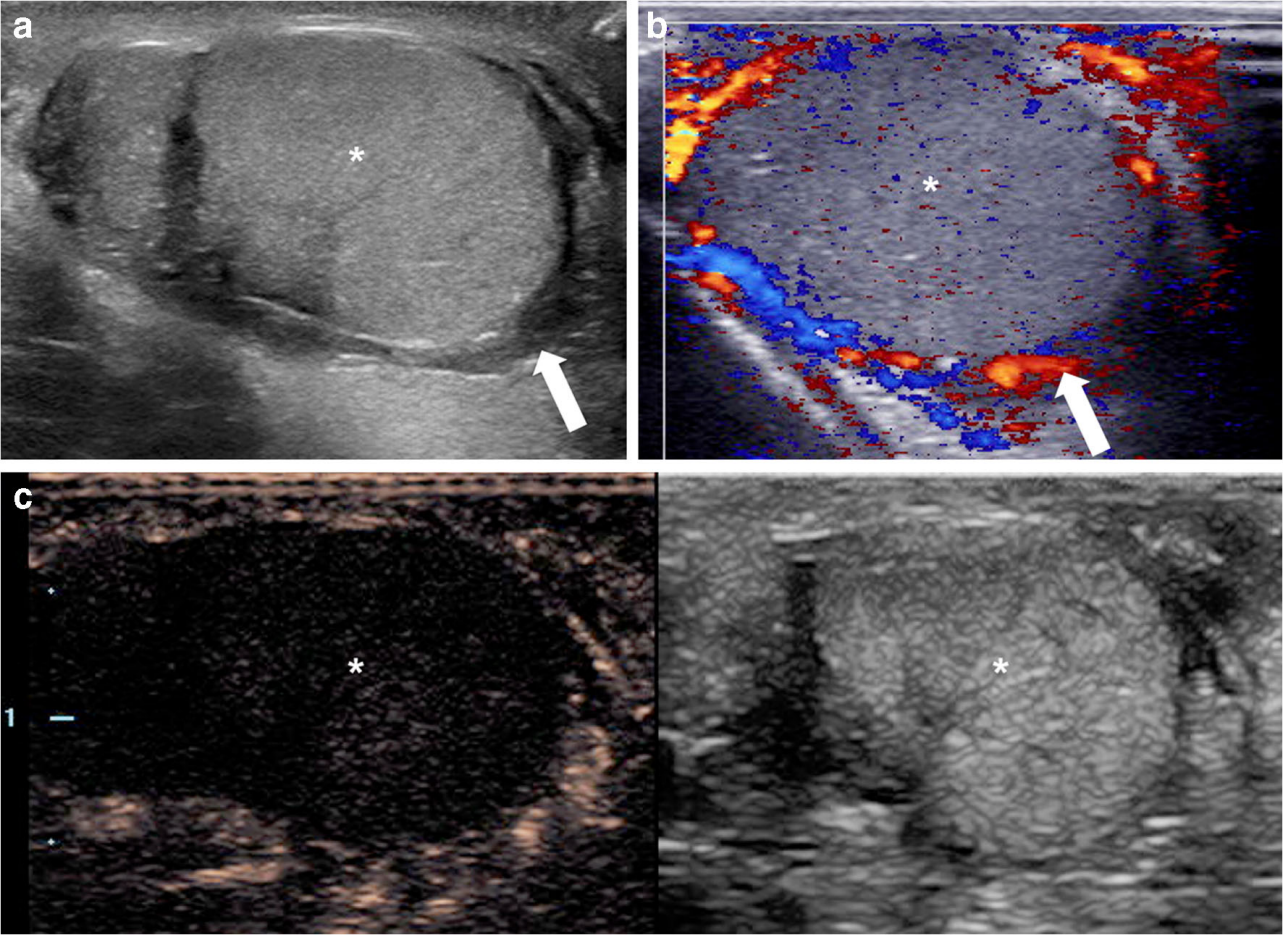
Acute scrotal pain in a 6-year-old boy with intellectual disability. a Longitudinal gray-scale US of the right testicle shows mild diffuse heterogeneity of the testicular parenchyma (*asterisk*). There is thickening and an edematous appearance of the scrotal wall (*arrow*). b Transverse color Doppler US image of the right testicle shows absence of color flow signal within the testicular parenchyma (*asterisk*). There is a rim of peripheral enhancement (*arrow*) corresponding to hyperemia of the scrotal wall. c Longitudinal dual display of contrast (*left*) and gray-scale (*right*) modes of the right testicle 49 s following contrast administration. There is complete absence of enhancement within the testicular parenchyma (*asterisk*), while contrast agent is seen in the surrounding tissues. See Online Supplementary Material 1 for cinematic images
